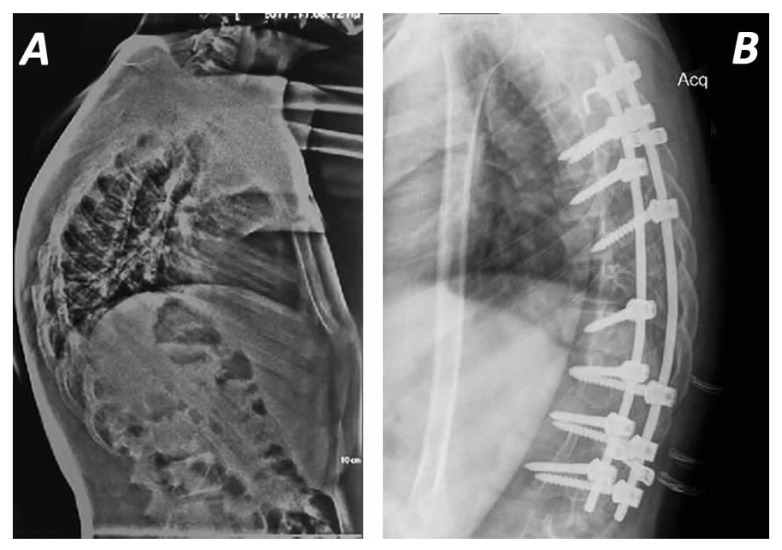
Pre- (A) and postoperative (B) radiographs of a 16-year-old male with Scheuermann’s disease. Posterior spinal fusion (T5–L1) was achieved with use of pedicle screws (T6, T7, T8, T10, T11, T12, L1), transverse process hooks (T5) dual (compression pre-curved) rod system and two cross-links. Fusion was enhanced with fresh frozen allograft (B). It must be highlighted that proximal bone hooks to prevent the Proximal Junctional Kyphosis (PJK) does not show any statistically significant difference compared to purely pedicle-screw-based constructs. The preoperative kyphotic deformity was 85° degrees extended from T2 to L2 (A), while after the spinal fusion between T2 to L2 was limited to 37° degrees (B).
